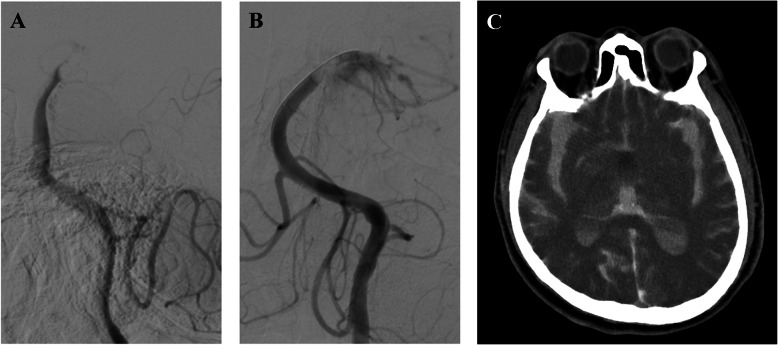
A 76-year-old man had a basilar artery occlusion. a, Angiography demonstrated complete occlusion of the distal basilar artery. b, After mechanical thrombectomy using a stent retriever, partial recanalization of the basilar artery was noted and extravascular contrast leakage was visible. c, Post-procedure CT showed subarachnoid and intraventricular hemorrhage
